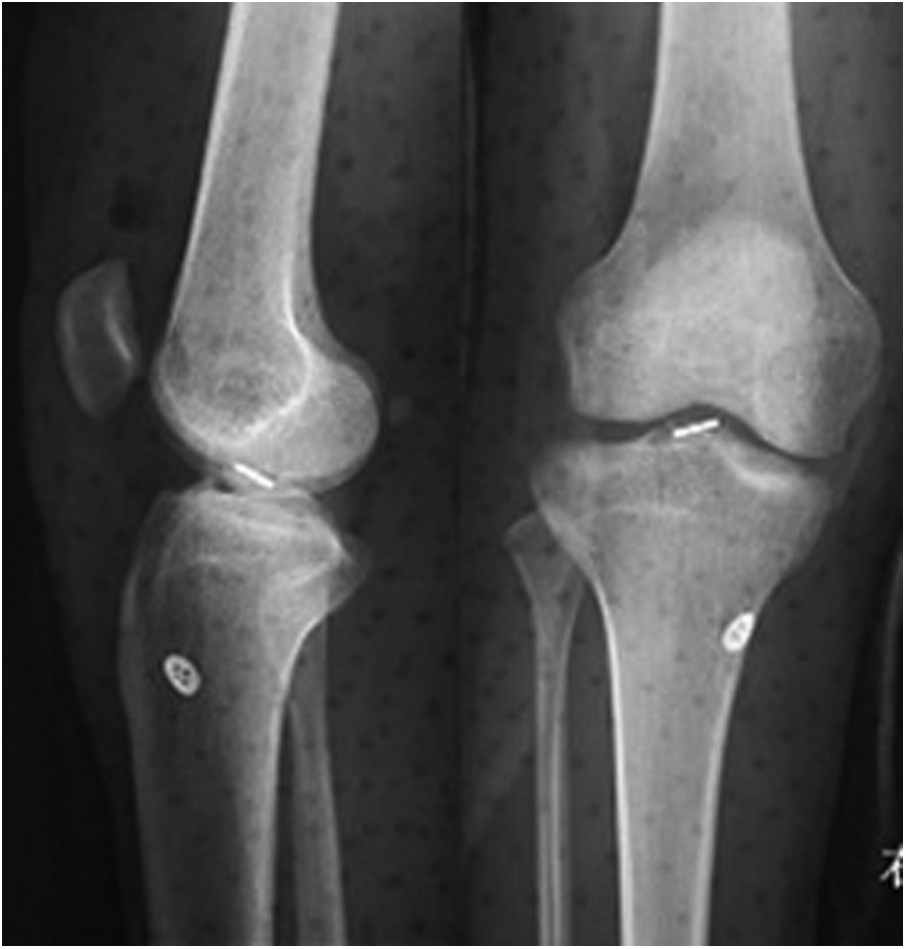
Postoperative anterior-posterior and lateral view radiographs. The nearly ideal position (B).
